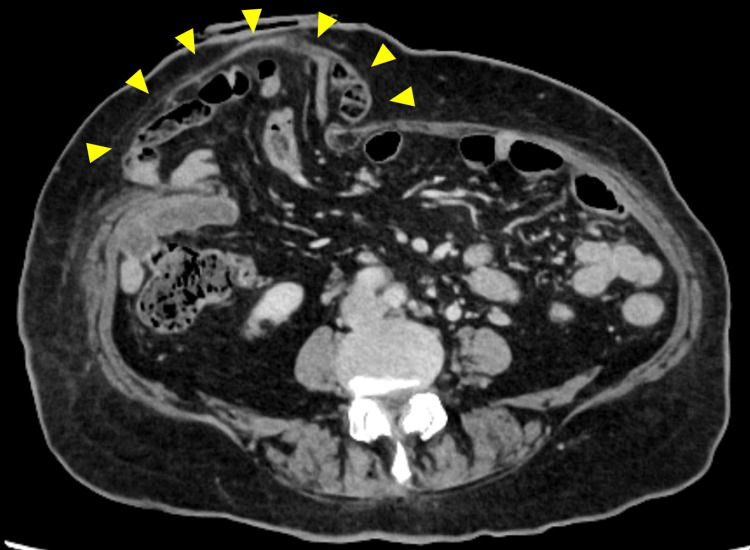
CT image of a parastomal hernia A large area of the bowel and mesentery (arrowheads) are herniated through the stomal orifice outside the abdominal wall. Image created by the authors
